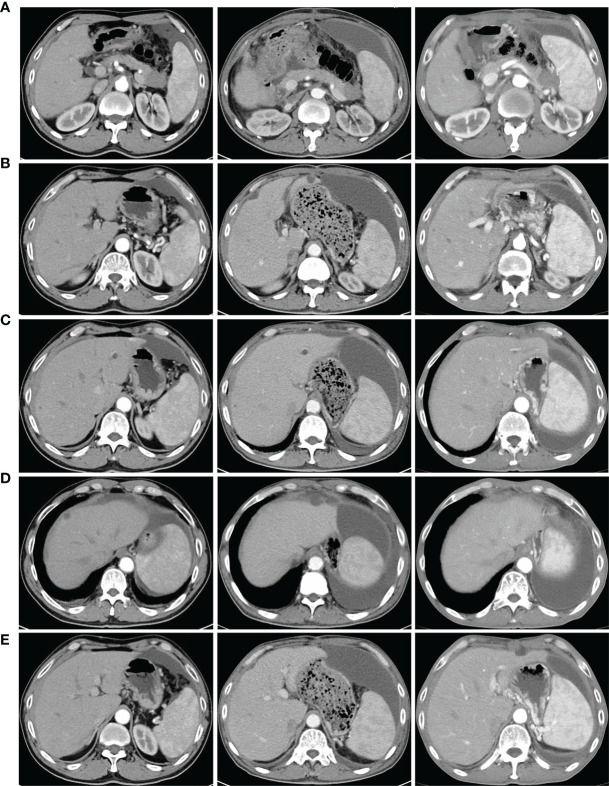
Contrast-enhanced CT of the whole abdomen and pelvic cavity of the patient in different periods. Enhanced scanning was performed using Omnipaque (300 mg/ml) with an injection rate of 3 ml/s. The arterial phase was scanned 28–30 s after injection, the portal phase 48–50 s, and the delayed phase 60–70 s. (A) Primary tumor: from left to right are preoperative, after chemotherapy, and after targeted treatment (the following sequence is from left to right). (B) No metastases before surgery, new metastases after chemotherapy, and metastases disappeared after target therapy. (C) Preoperative metastases, metastases increased after chemotherapy and decreased after targeted therapy. (D) Preoperative metastases, new metastases after chemotherapy and disappeared after targeted therapy. (E) No metastasis here before surgery, no metastasis here after chemotherapy, and new recurrence after targeted therapy.
